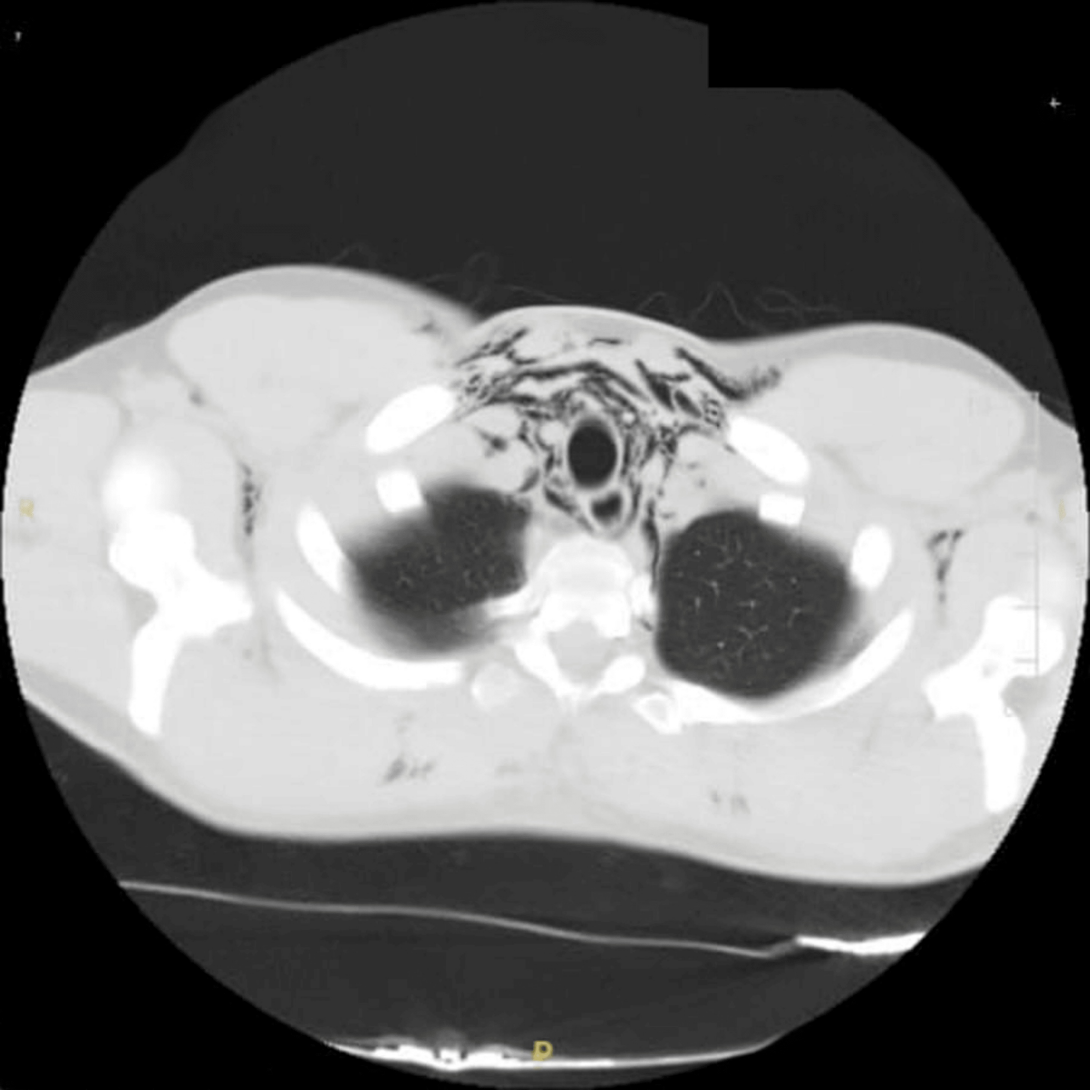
Computed tomography (CT) of the thorax showing air within the mediastinum.
